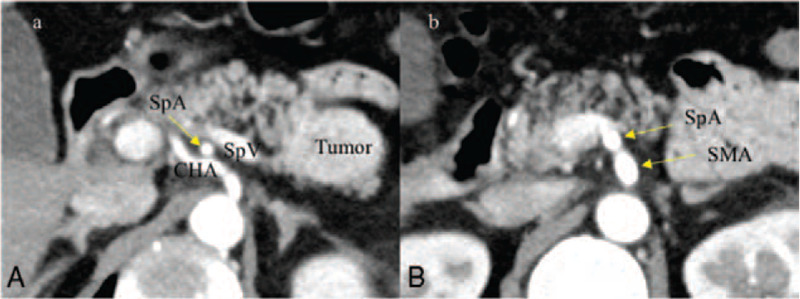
Preoperative CT images. A: A high enhanced tumor measuring 45 mm in a diameter at the pancreatic tail was detected. SpA was running near the root of CHA. B: SpA originating from SMA was running dorsally to the pancreas. *CHA* = common hepatic artery, *SpA* = splenic artery, *SpV* = splenic vein.
